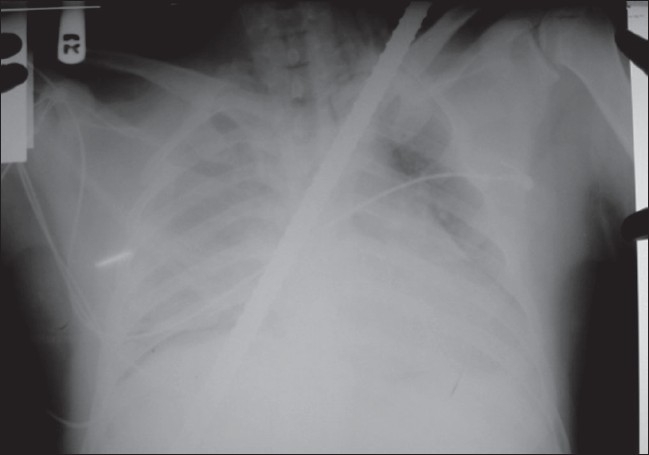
Chest X-ray showing metallic bar
